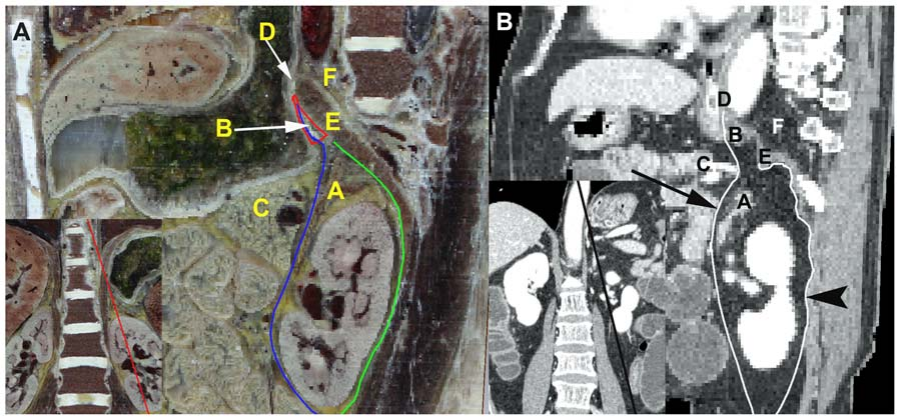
Manifestation of the spatial relationship between the LES and left retroperitoneal space on oblique sagittal planes. (A, B) Visualization of the communication relationship between the left retroperitoneal space and the LES from the lateral superior view on CVH2 and CT images, respectively. The anterior renal fascia is represented as a blue line and labeled by a black arrow on CVH2 and CT images, respectively. The posterior renal fascia is represented as a green line and labeled by an arrowhead on CVH2 and CT images, respectively. A: left adrenal gland; B: LES; C: pancreas; D: esophageal hiatus; E: left diaphragm; F: retrocrural space.
